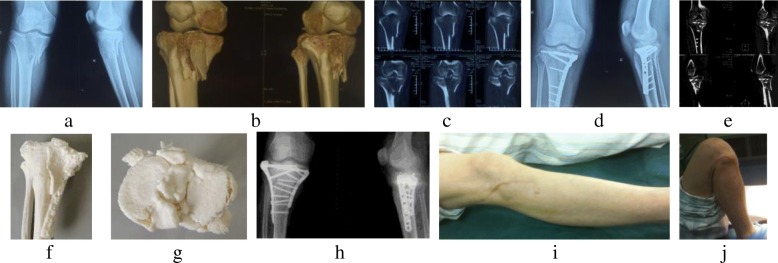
A 54-year-old male patient sustained the tibial plateau fracture caused by bicycle accident (Schatzker V, AO/OTA 41.C1). The first preoperative X-rays in anteroposterior and lateral views (a) and CT-scans (b, c) showed the tibial plateau fractures of medial and lateral condyle and the slight collapse of the lateral articular surface. The second preoperative X-rays in anteroposterior and lateral views (d), CT scans (e), and 3D printing model (f, g) showed the widening of the tibial plateau, the collapse of the lateral articular surface, and the poor reduction of the medial plateau. The X-rays in anteroposterior and lateral views (h) after the revision surgery indicated the correction of the widening of the tibial plateau, the restoration of lateral articular surface, the satisfied reduction of the medial plateau, and the favorable internal fixation of the anterolateral and posteromedial plate. The functional appearance (i, j) at 30 months after the revision surgery showed that the nice healing of the wounds and the satisfactory function of THE knee joint
